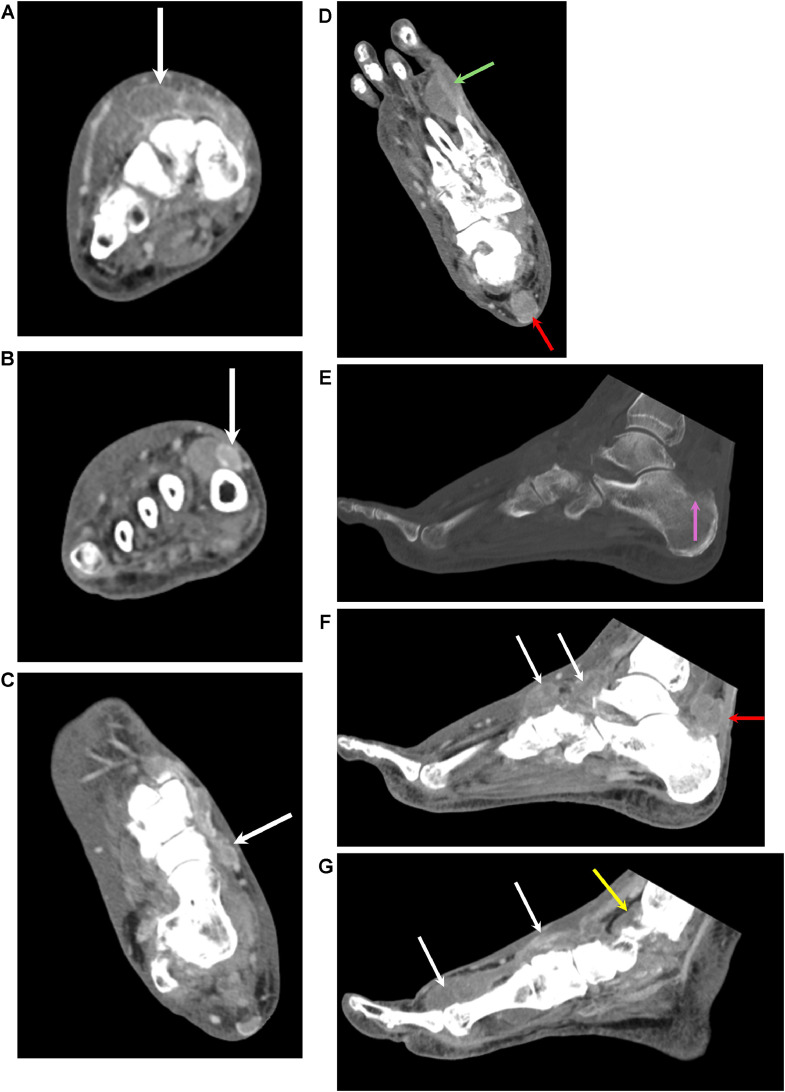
(A and B) Short axis CT of the right foot with IV contrast shows areas of peripherally enhancing soft tissue/fluid collections at the dorsal aspect of the first/second rays (white arrows). There is diffuse subcutaneous edema. (C and D) Long axis CT of the right foot with IV contrast shows areas of peripherally enhancing soft tissue/fluid collections along the first ray (white arrows). This is likely associated with the extensor hallucis longus tendon sheath (green arrow). There is abnormal soft tissue deep to the Achilles tendon (red arrow). There is diffuse subcutaneous edema. (E-G) Sagittal CT of the right foot with IV contrast shows subtle erosion of the posterior superior calcaneus (pink arrow). There are multiple areas of peripherally enhancing soft tissue/fluid collections, including deep to the Achilles tendon (red arrow) and dorsal foot (white arrows). There is an effusion or synovitis in the ankle joint (yellow arrow). There is diffuse subcutaneous edema.
